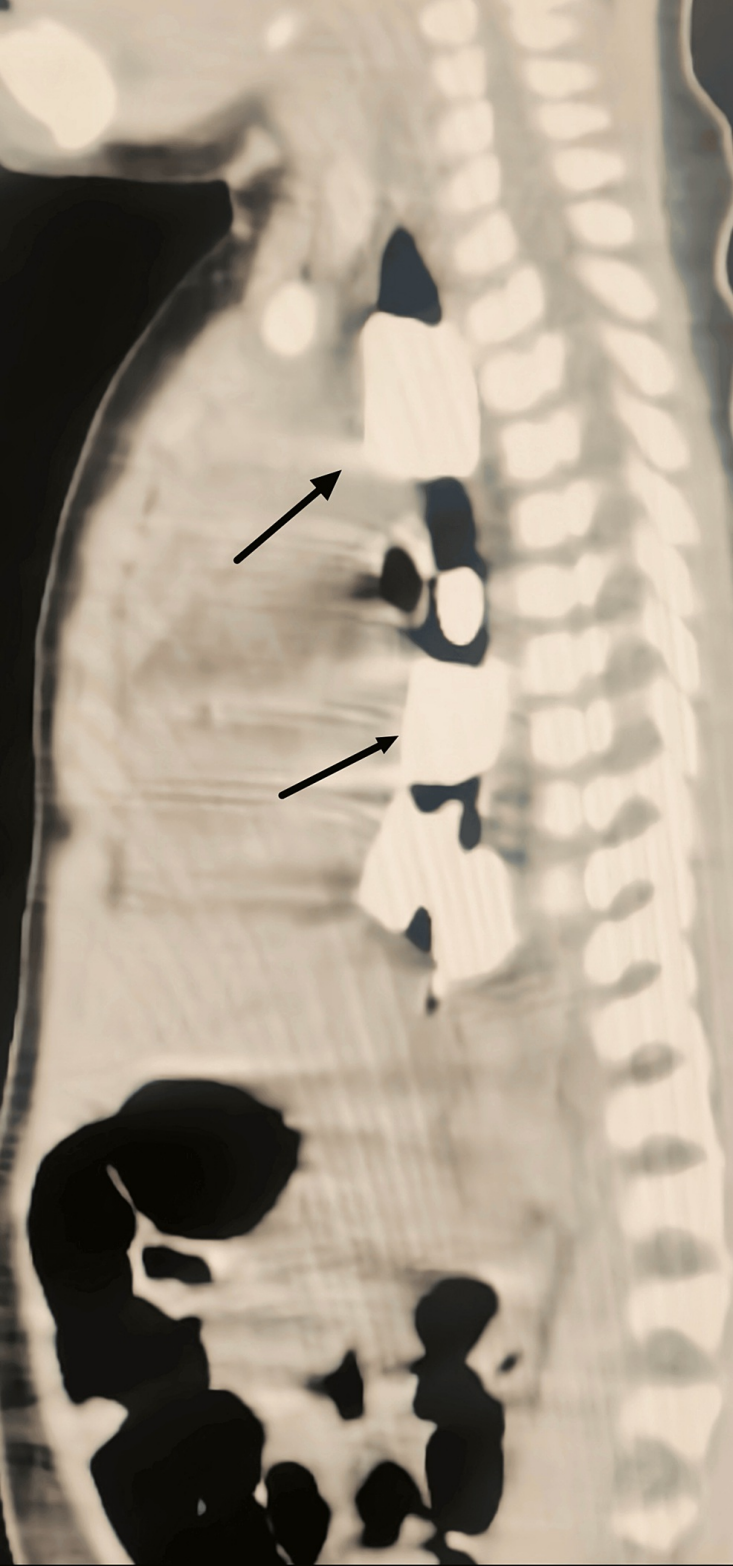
CT thorax image of the metallic foreign bodies
